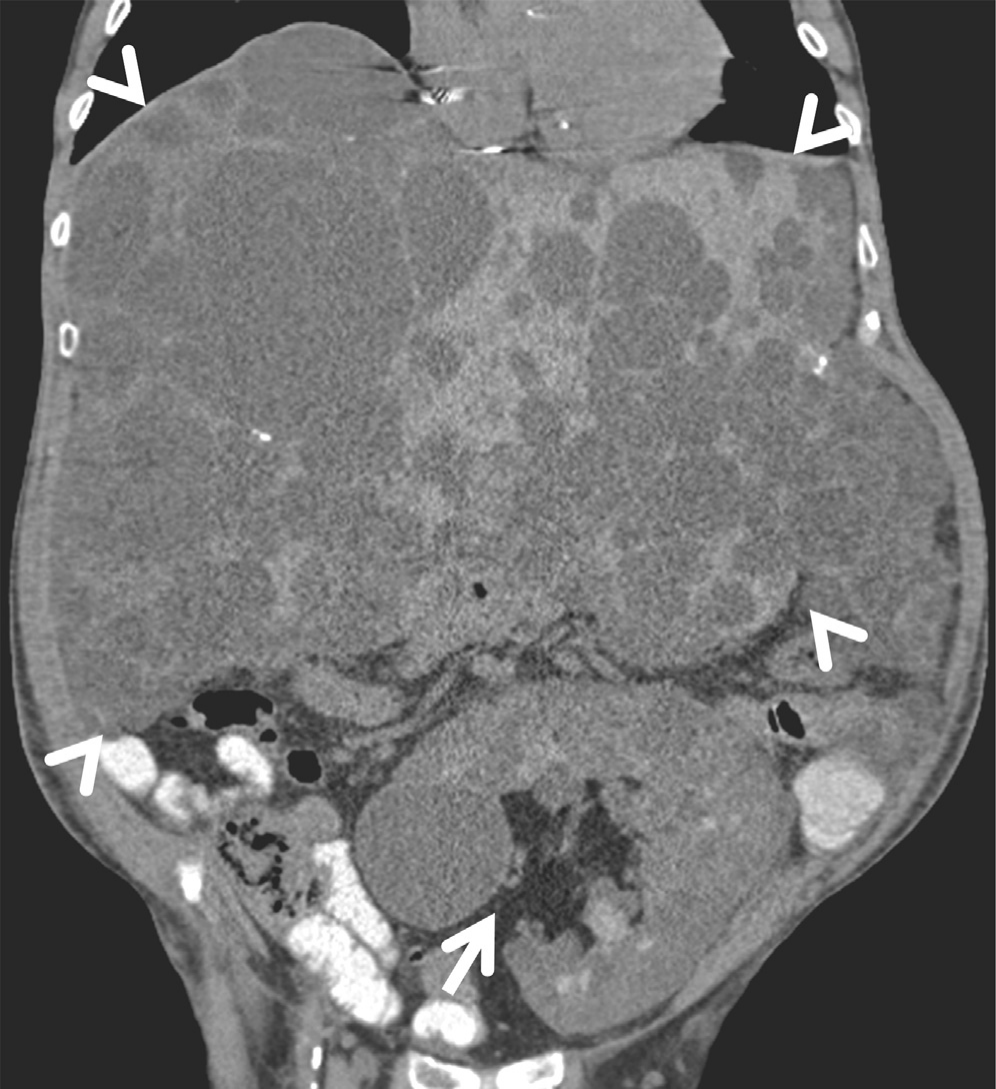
A computed tomographic scan of the abdomen without intravenous contrast shows severely enlarged polycystic liver (arrowheads) and a polycystic kidney transplant in the left lower quadrant (arrow). The cystic allograft volume is 1244 ml.
